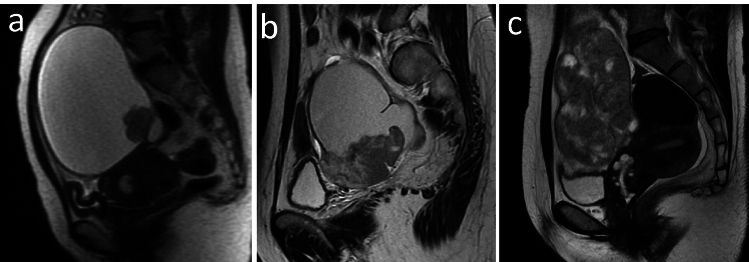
Configuration of the MRI-based features in ovarian carcinomas. a Predominantly cystic (≤ one-third solid component) in clear cell carcinoma on single-shot fast-spin echo (SSFSE) T2-weigthed image (T2WI), b solid and cystic (one-third to two-thirds solid component) in endometrioid carcinoma on fast-spin echo T2WI, and c predominantly solid (≥ two-thirds solid component) in endometrioid carcinoma on fast-spin echo T2WI
